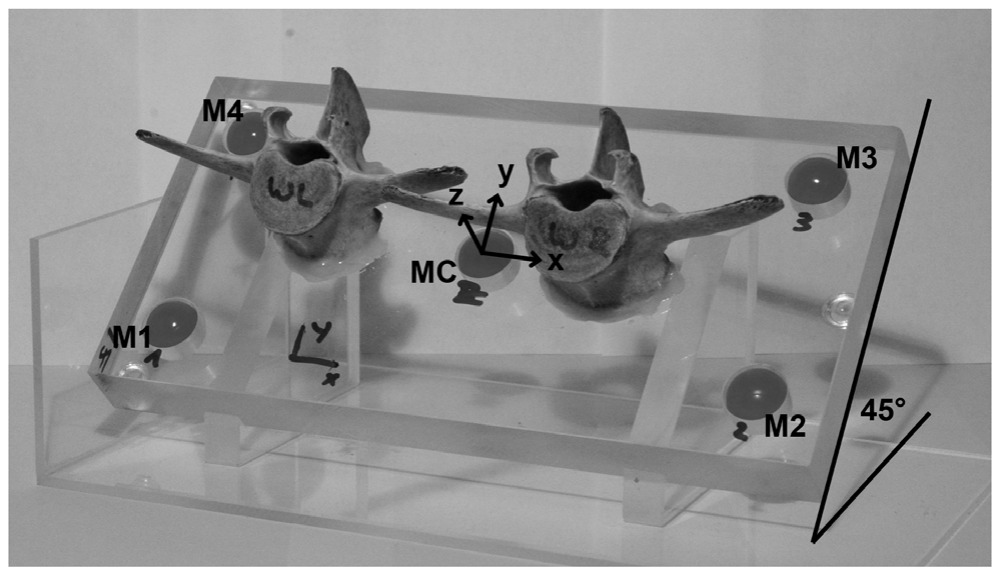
Acrylic glass plate with five MRI visible markers (paintballs: M1, M2, M3, M4, MC, with distances of M1–M2 = 180 mm, M2–M3 = 70 mm) and two lamb vertebrae, shown in the forward tilted position (45°).
